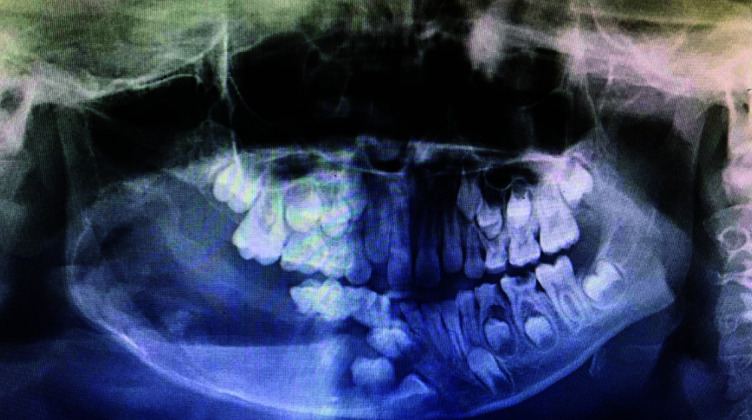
Post-operative panoramic radiograph at 8 months of follow up
